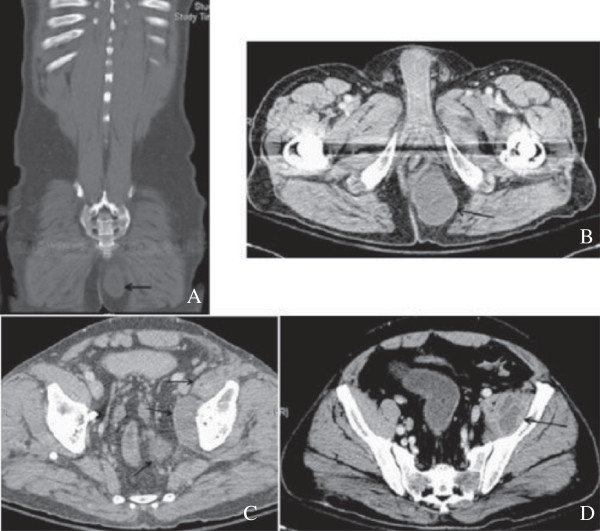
Magnetic resonance imaging of the pelvis demonstrated large, complex, multiple lobulated abscesses with lower central attenuation and peripheral enhancement from the left ischiorectal fossa to the left iliacus muscle, iliopsoas muscle, and adductor muscle. (A, B) One large complex abscess in the ischiorectal fossa with mass effect to the rectum. (C, D) Abscess extending to the iliopsoas muscle and iliacus muscle with multiple loculation.
